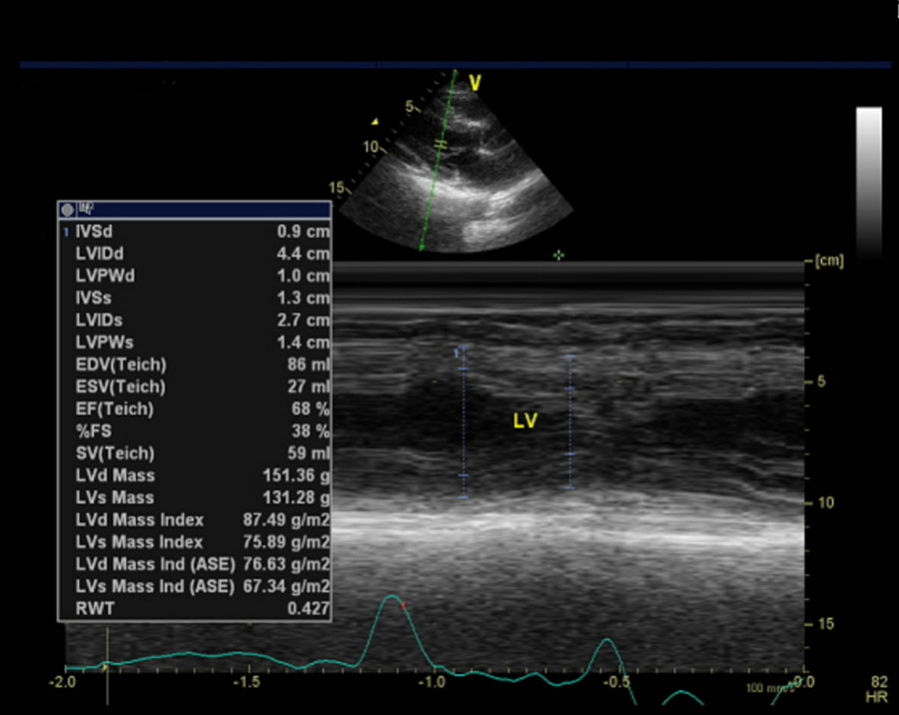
Echocardiography of third week after hospitalization.
